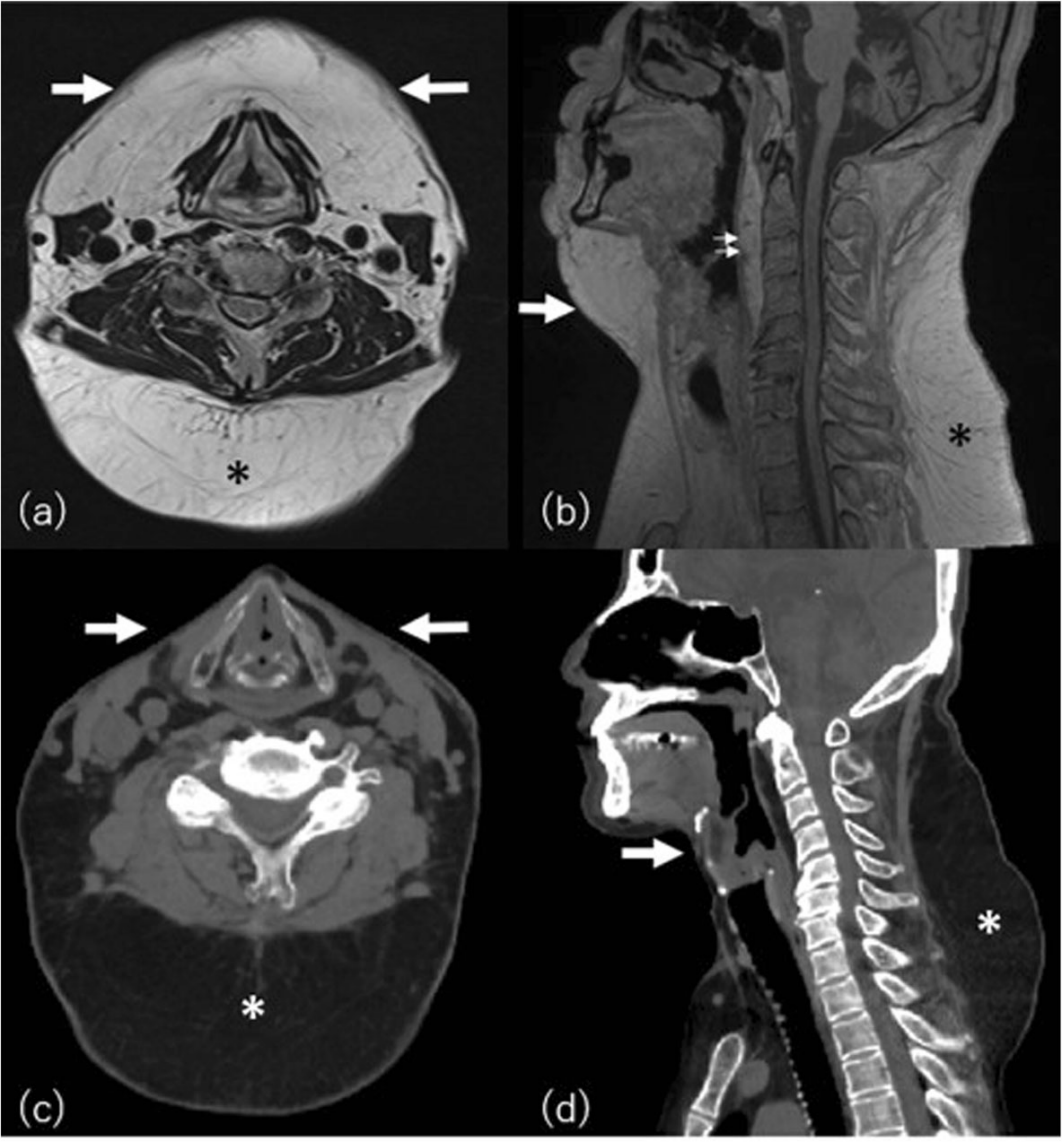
A 45-year-old man with multiple symmetrical lipomatosis with sleep apnea. a Axial T2-weighted and (b) T1-weighted image show abnormal subcutaneous fat deposition in the anterior (arrows) and posterior neck (asterisks). Note the increase of adipose tissue also observed in the posterior pharyngeal space (small arrows). c Post liposuction axial and (d) sagittal reconstructed CT 2 years later. Subcutaneous fat volume in the anterior neck is markedly decreased (arrows), whereas in contrast, the fat deposition in the posterior neck has worsened (asterisk)
